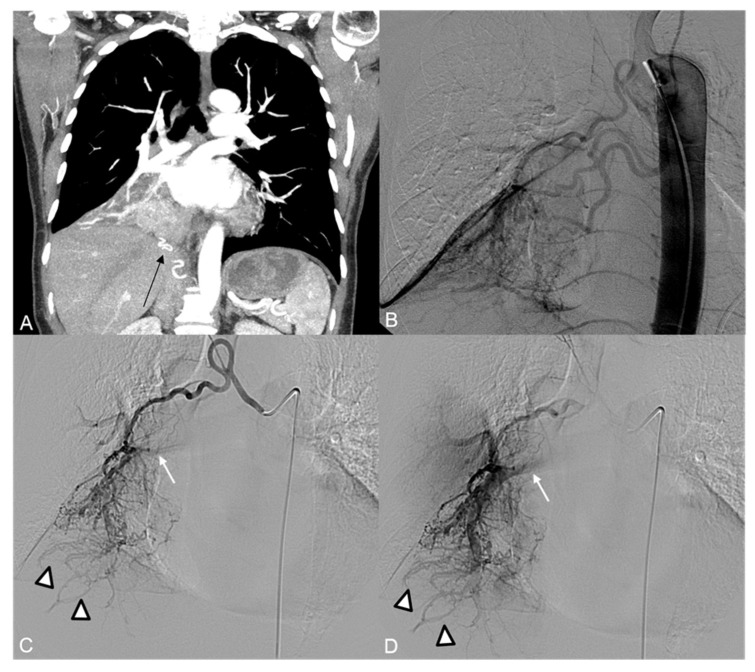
(A) CT Angiography, coronal view: highly vascular mass inferior to the right hilum. Hypertrophic right phrenic artery ascending to the mass (arrow). (B) The preliminary aortography shows the presence of three hypertrophic bronchial feeders to the tumor with intralesional fistulae between bronchial arteries and the inferior pulmonary veins. (C,D) Selective angiogram of the superior right bronchial artery in different consecutive phases shows the recruitment of enlarged bronco-pulmonary anastomoses (arrowhead), with early opacification of the inferior pulmonary vein (arrow).
